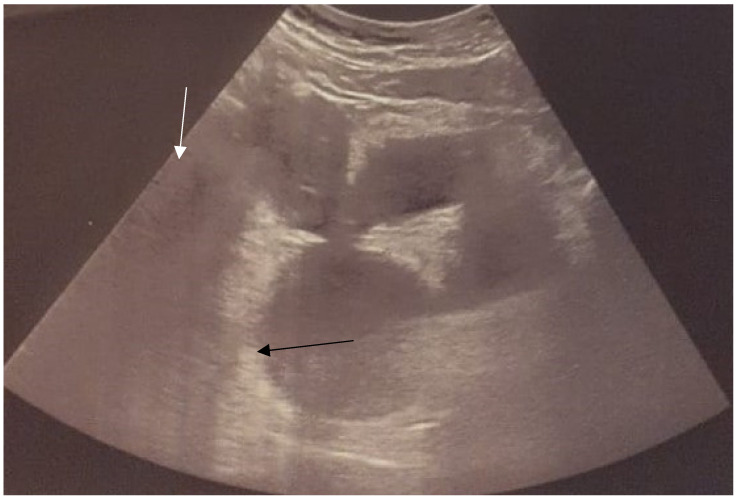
Ultrasound from extern hospital, when the diagnosis of hematocolpos was mistaken as an ovarian cyst (black arrow). Double uterus was not recognized (white arrow).
